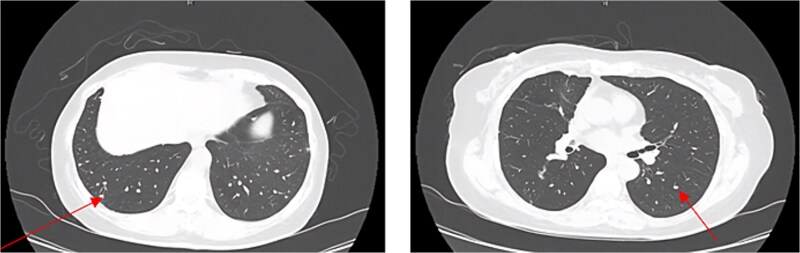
CT chest showing multiple bilateral nodule.
